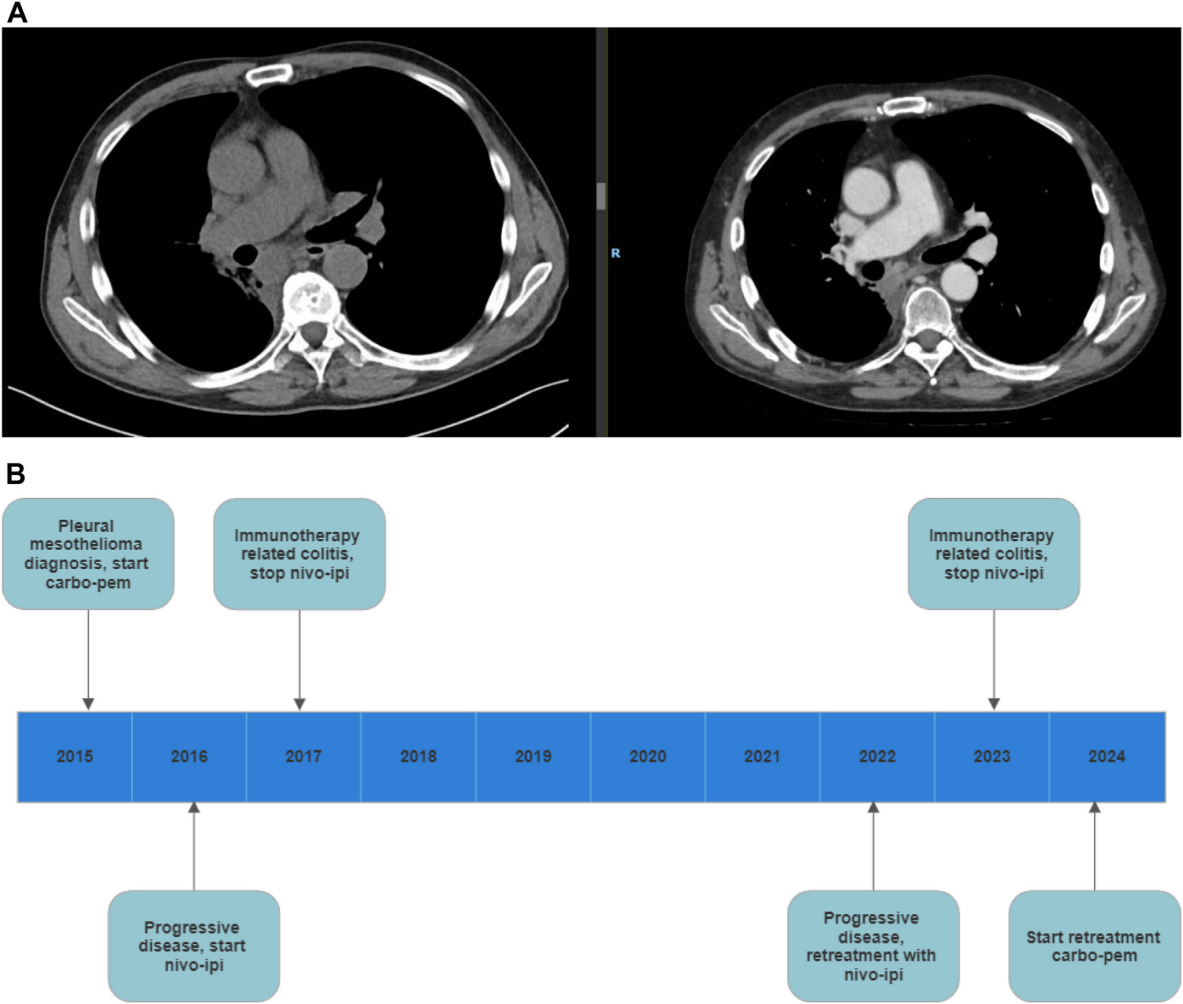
(*A*) Case 1: CT scan (without contrast) 2 weeks before start of retreatment with nivo-ipi (left) and CT scan (with contrast) 9 weeks after start of retreatment with nivo-ipi (right). (*B*) Case 2: Timeline of events. Carbo-pem, carboplatin-pemetrexed; CT, computed tomography; nivo-ipi, nivolumab-ipilimumab.
